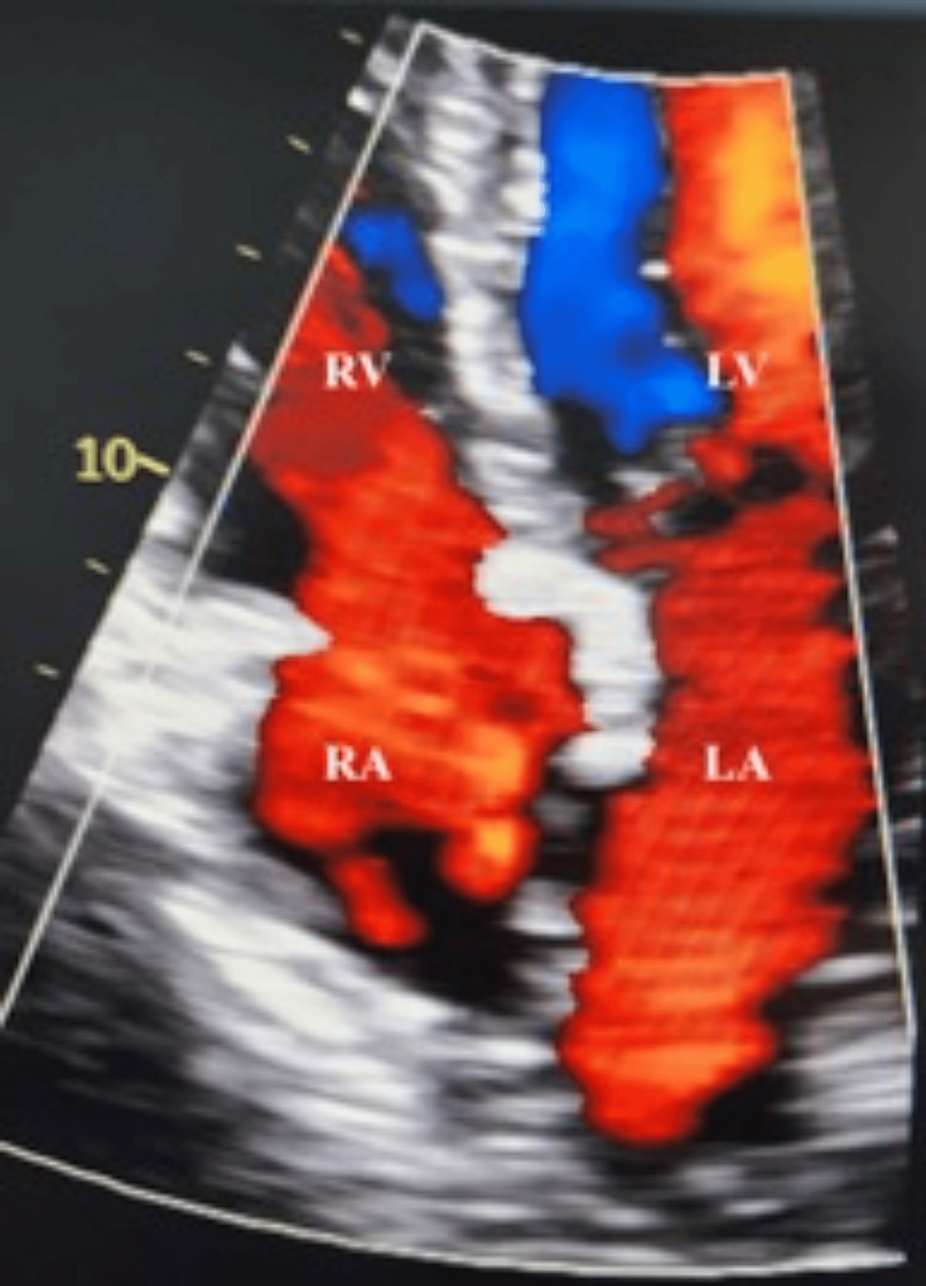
Post-closure echocardiogram This is a four-chamber view showing postoperative atrial septal defect (ASD) repair, tricuspid valve repair, and left atrial appendage ligation. As noted in the image, there is no mixing of color across the atrial septum confirming the successful closure of ASD. LA, left atrium; LV, left ventricle; RA, right atrium; RV, right ventricle.
